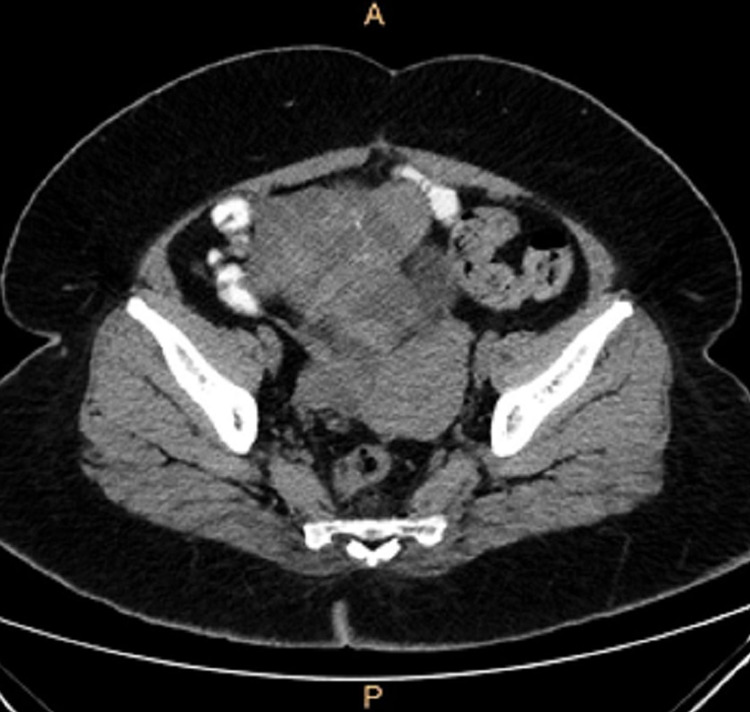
CT axial image - no fat density, calcification, no free fluid, lymphadenopathy and mesenteric nodules
